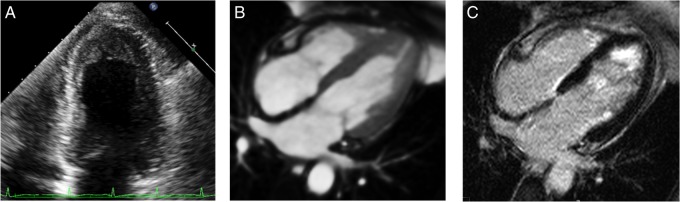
(A) Two-chamber echocardiogram. (B) Four-chamber steady-state free precession cardiac MRI. (C) Phase-sensitive inversion recovery cardiac MRI following gadolinium contrast.
